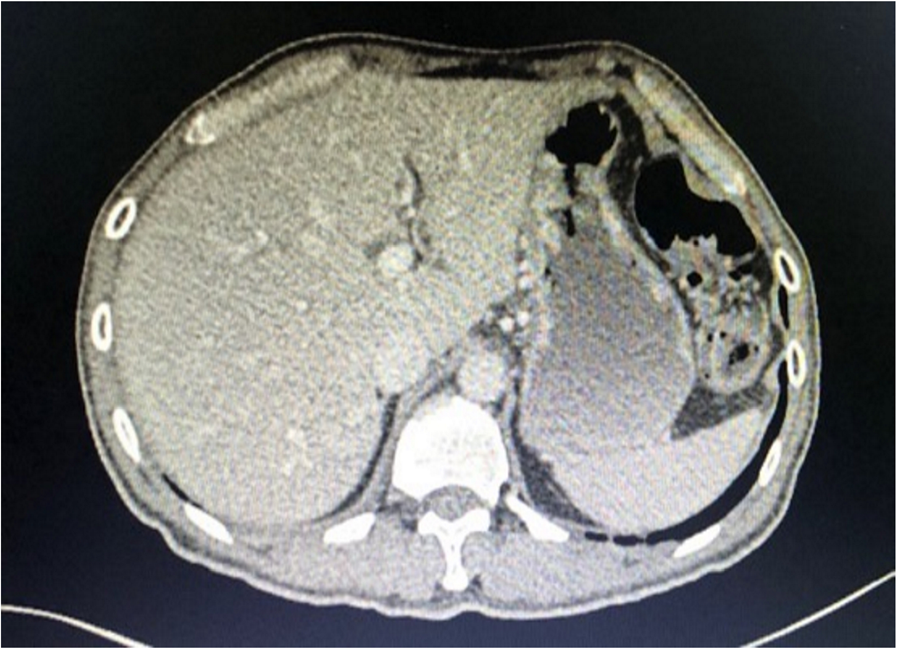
Resolution of TB liver on CT imaging after 6 months post ATT
